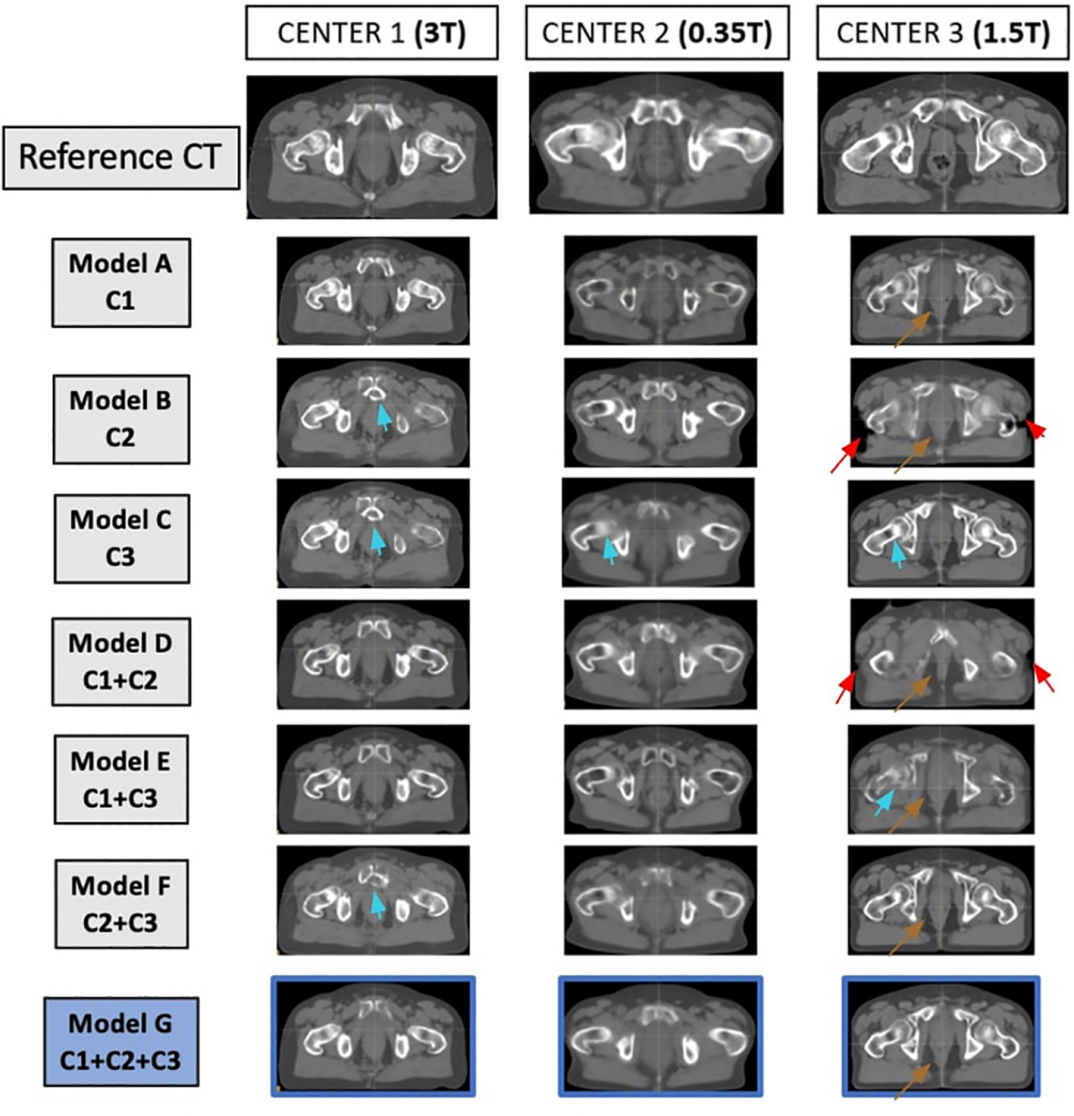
Matched reference CT and synthetic CT (sCT) for each model, for one patient at each center. On the first line, from left to right, the reference CTs of one patient from center 1, one patient from center 2, and one patient from center 3 are represented. For each patient in each center, the sCT generated with each model is represented. The sCT images of each model represent the same slice as the reference CT. Arrows show limitations of the sCT generation (red arrow for misgeneration of the external contour, brown arrow for misgeneration of air pockets, blue arrows for misgeneration of bones).
